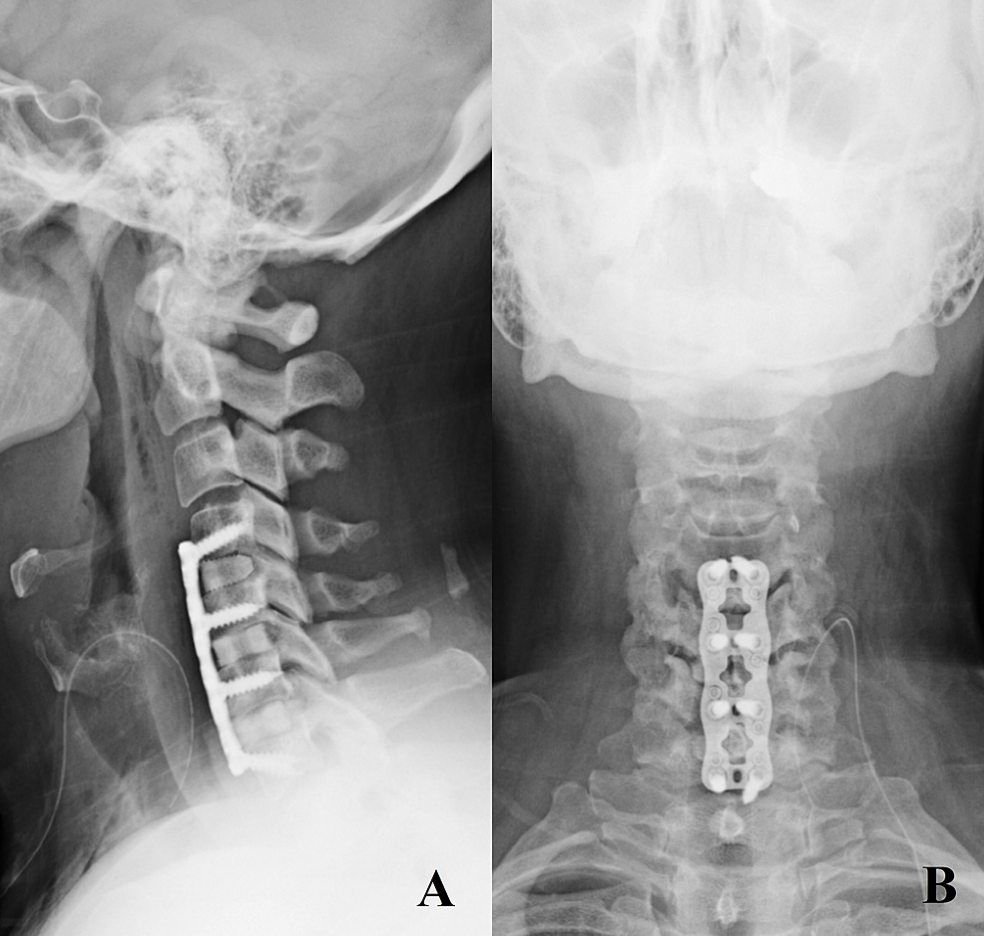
Postoperative x-ray after C4-7 ACDF with “kissing” allografts at C5-6, C6-7. (A) lateral and (B) AP views demonstrating prior graft placement on postoperative day one. A single allograft interbody was used at C4-5.
